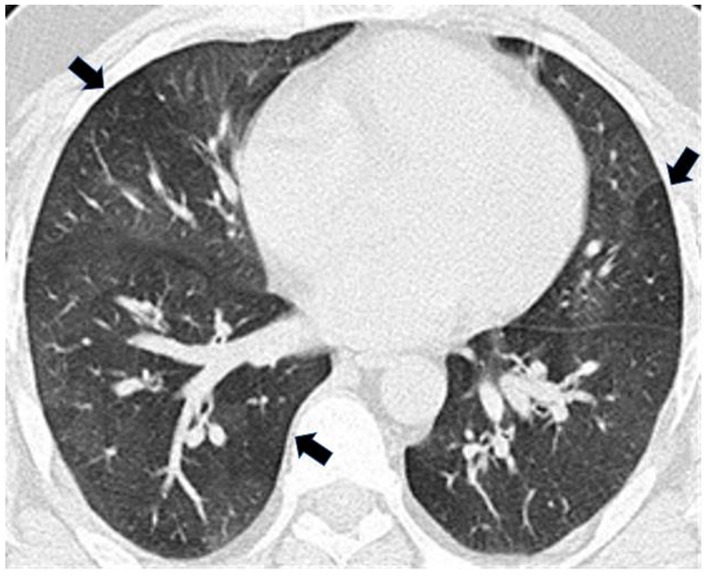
Expiratory high-resolution computed tomography (HRCT) revealed bilateral mosaic attenuation (arrows) consistent with air trapping.
